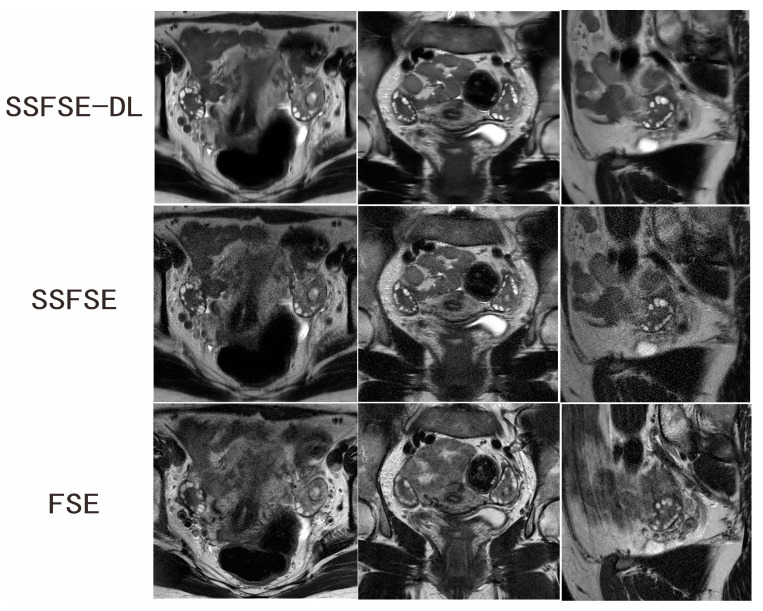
Ovarian MRI in a 28-year-old woman with confirmed PCOS. SSFSE-DL images (upper row) show the least noise and blurring artifacts. The bilateral enlarged ovaries with many small peripheral follicles are clearly delineated on the SSFSE-DL images. The display of the follicles is impaired by the noise on the SSFSE images (middle row) and by the blurring artifacts in the FSE images (lower row).
